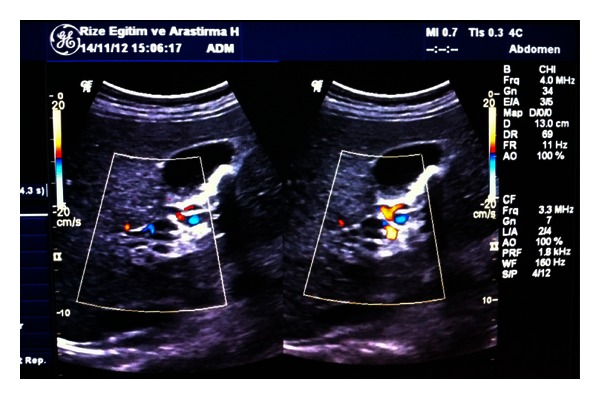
Multiple serpiginous venous collaterals with turbulent venous flow around the thin thrombosed portal vein consistent with cavernous transformation of the portal vein can be seen in the color Doppler ultrasound image.
